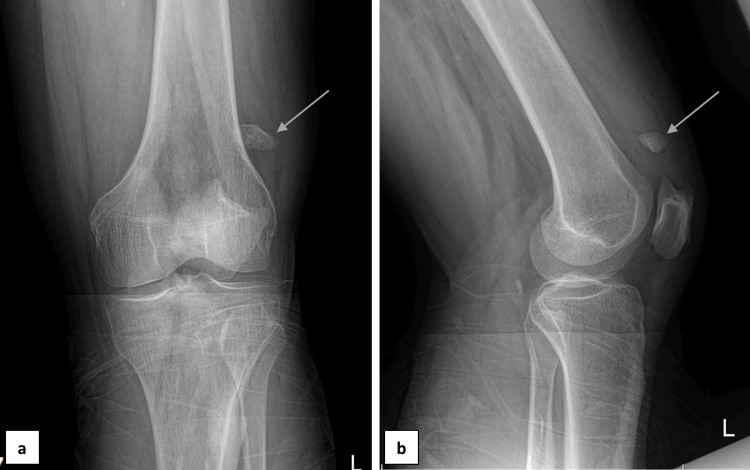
X-ray images of the left knee Anteroposterior (a) and lateral (b) X-ray views of the left knee indicating separation of Saupe Type III bipartite patella. The arrows indicate the separated anterosuperior patellar fragment.
